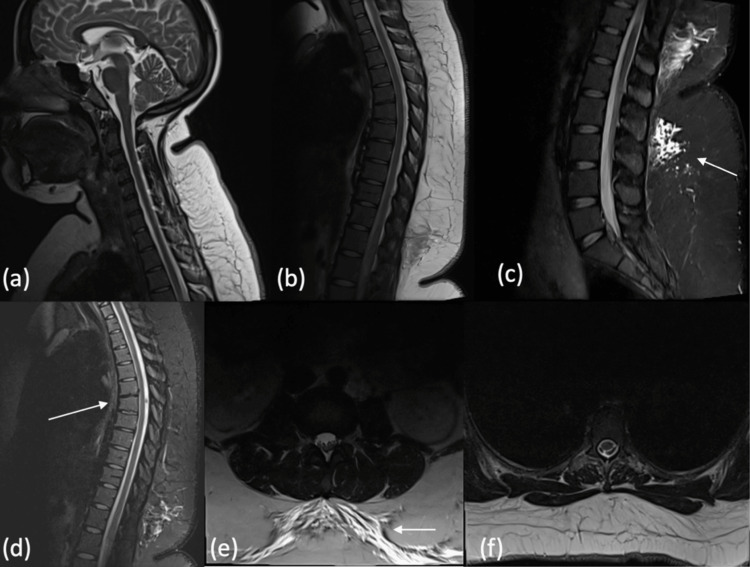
Whole Spine MRI Without Contrast (a) sagittal cervical spine with preserved alignment, canal diameter, and cord signal; (b) sagittal thoracic spine with no abnormal findings; (c) sagittal lumbar spine with no abnormal findings; (d) mild anterior wedging of the D6 vertebra; (e) small areas of lumbar subcutaneous edema, likely related to epidural puncture; (f) axial lumbar section showing preserved cord signal and absence of compression.
